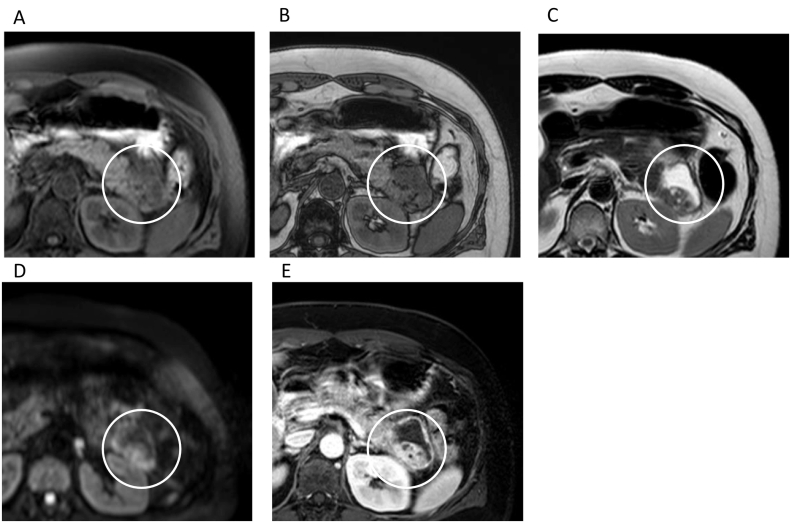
A; T1WI in-phase, B; T1WI opposed-phase, C; T2WI, D; DWI, E; Dynamic early phase. MRI showed the lesion had a solid component which was low intensity in T1WI, high intensity in T2WI and DWI. The nodule was enhanced in the early phase, and had microcystic lesions inside.
